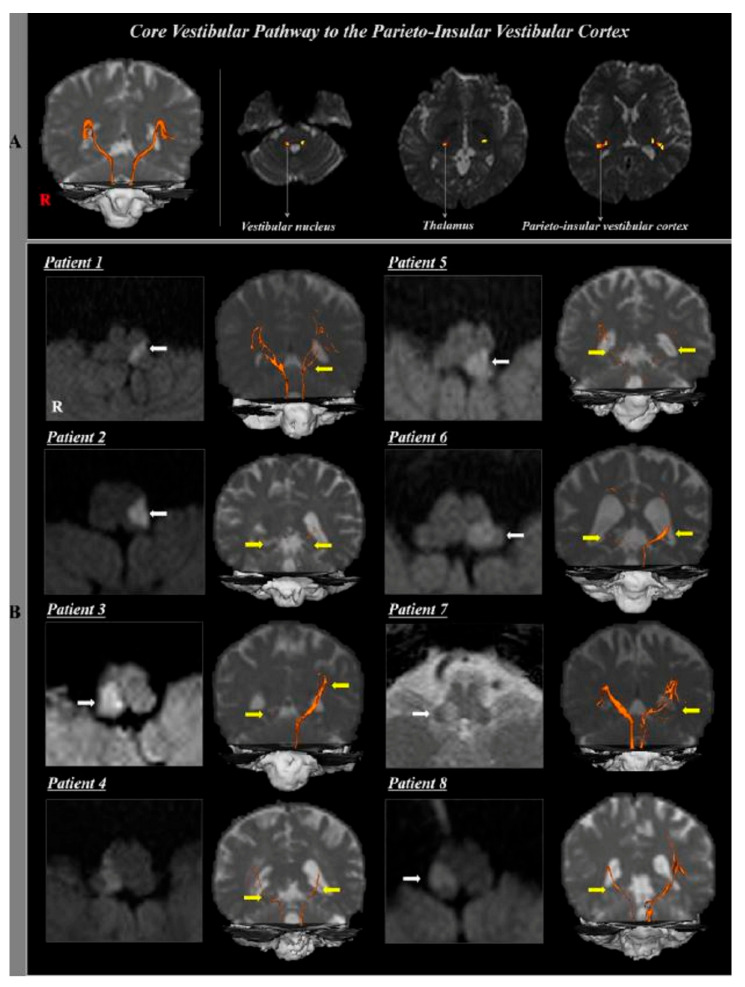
Anatomical structure of CVP captured using DTT [39]. (A) Core vestibular pathway to the PIVC in normal control subject (53-year-old male) (B) Computed tomography (CT) and diffusion tensor tractography (DTT) of the core vestibular pathway to the PIVC in eight patients with dorsolateral medullary infarct (white arrow).; bilateral or unilateral core vestibular pathway to the PIVC were injured due to dorsolateral medullary infarct (yellow arrow). Copyright by Yeo et al. (2018) [39].
